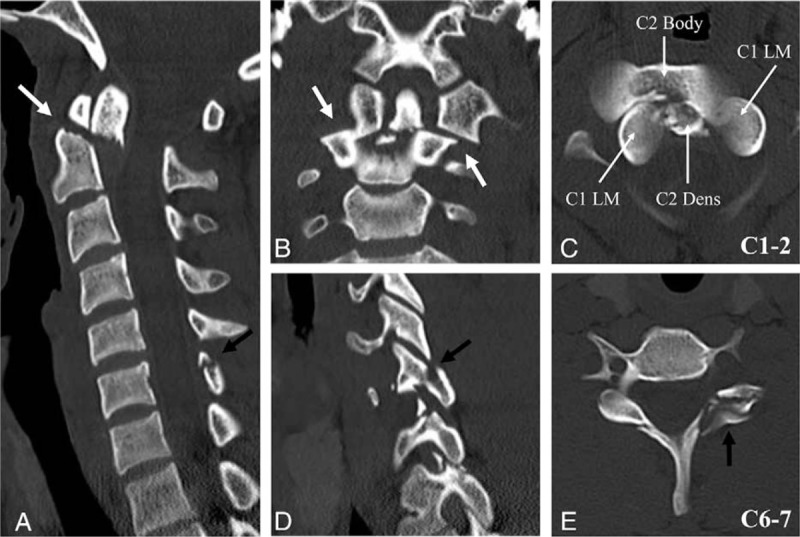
Computed tomography (CT) scans showing posterolateral dislocation of C1 on C2 (white arrows) with type II dens fracture (Anderson and D’Alonzo classification) (A, B, and C), unilateral facet fracture, and subluxation of C6–7 (black arrows) (D and E).
